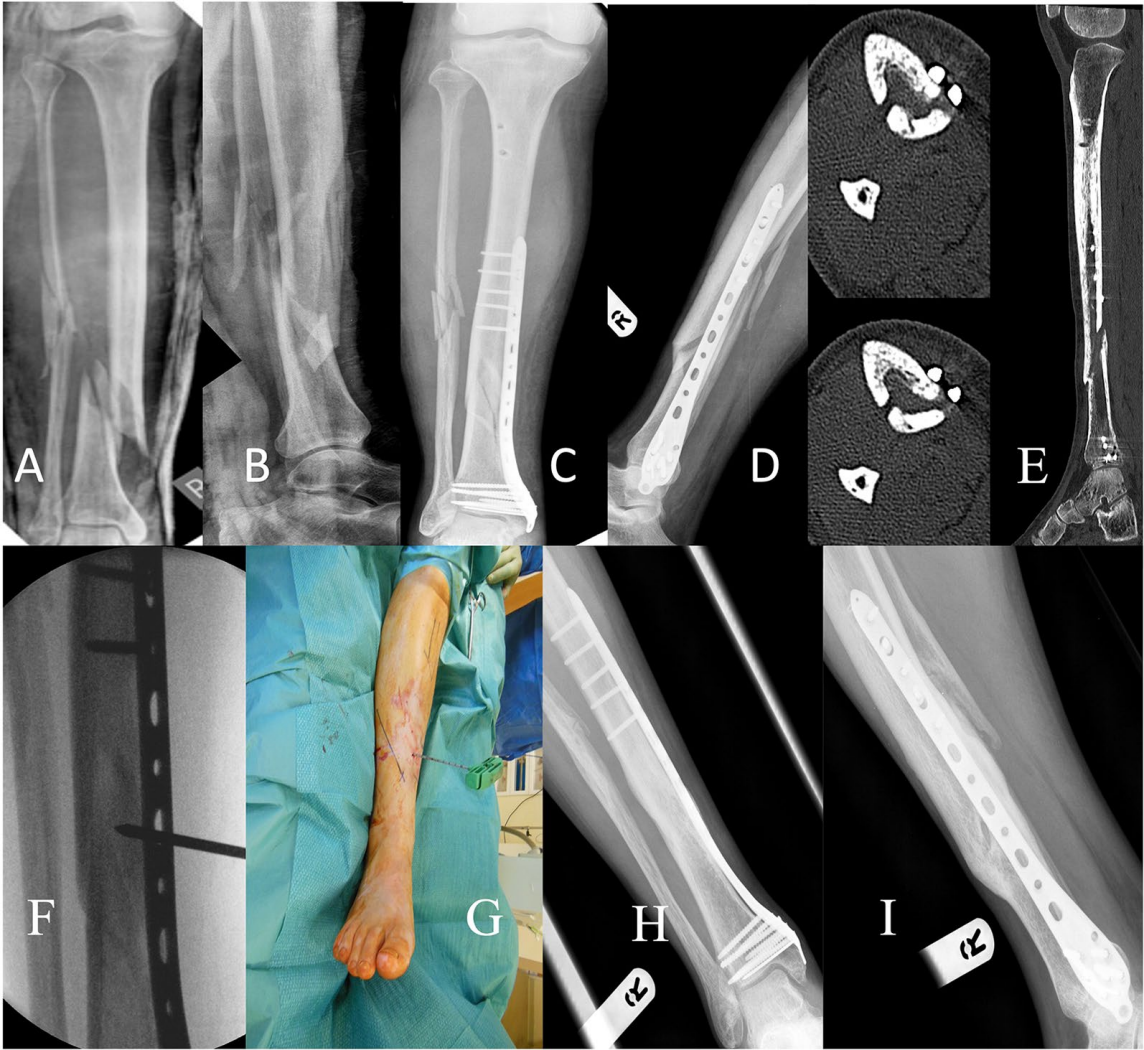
A 30-year-old female presented a CT-proven atrophic nonunion at 9 months following an IM nail for a closed femoral fracture (A, B). The patient underwent the harvesting of 60 ml of bone marrow, which was concentrated down to 8 ml volume and injected into the nonunion site (C, D). Images E and F demonstrate union at 3 months post-operation. *IM* Intramedullary
